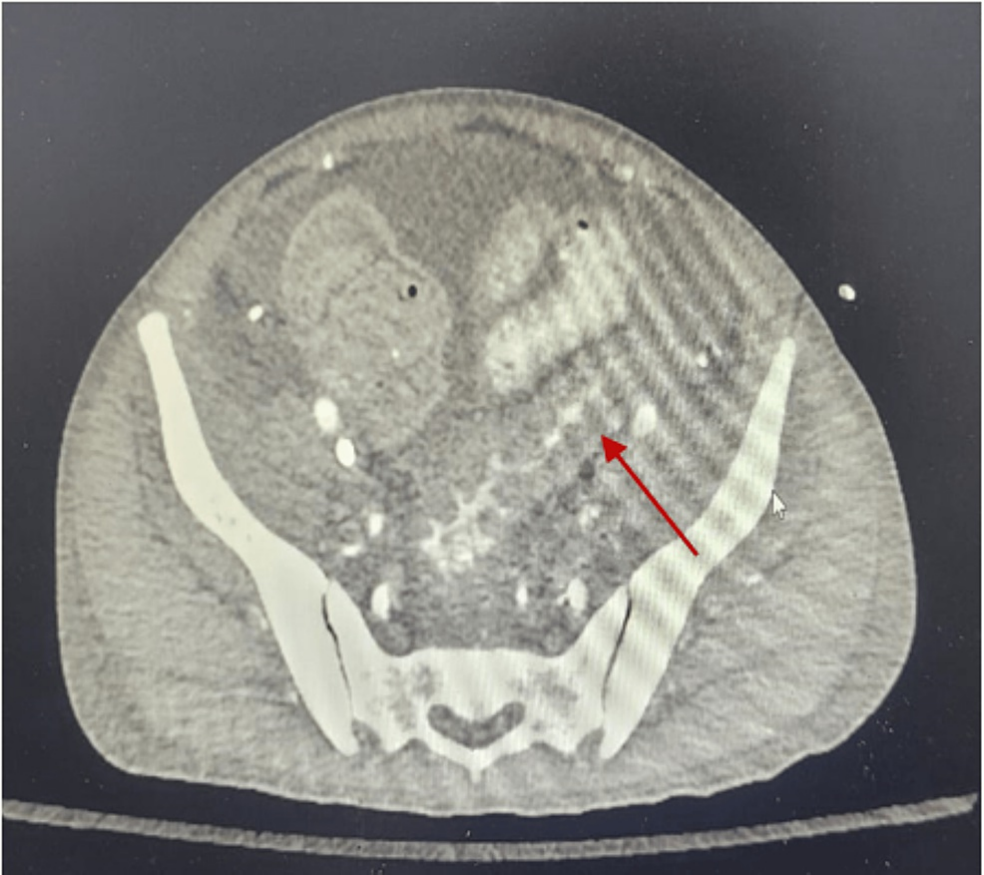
Contrast-enhanced computed tomography axial section showed thickening of peritoneal lining suggestive of peritonitis, colitis of distal large bowel and rectum with no signs of bowel perforation.
